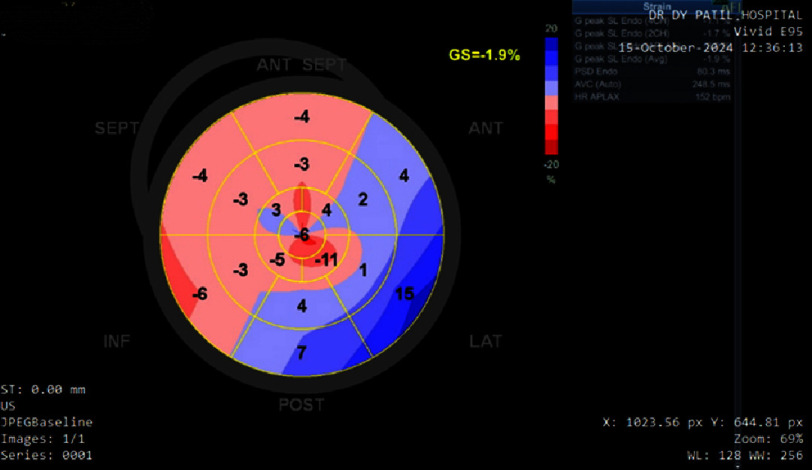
Follow-up speckle-tracking transthoracic echocardiographic global longitudinal strain (GLS) bull’s-eye plot demonstrating severely impaired myocardial strain with a calculated GLS of −1.9%. The segmental strain map shows diffuse reduction in longitudinal deformation with loss of the previously observed apical sparing pattern, consistent with interval progression of myocardial involvement compared with the baseline study.
